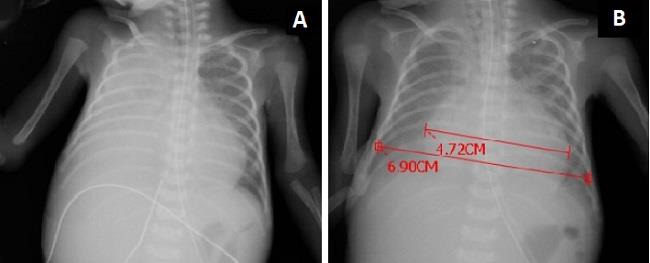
A. Chest radiograph shows right-sided pleural effusion. Note the catheter tip was in correct position. B. Chest radiograph after right thoracentesis. Catheter was removed. Lung fields were clear. Cardiothoracic index increased (0.66).
